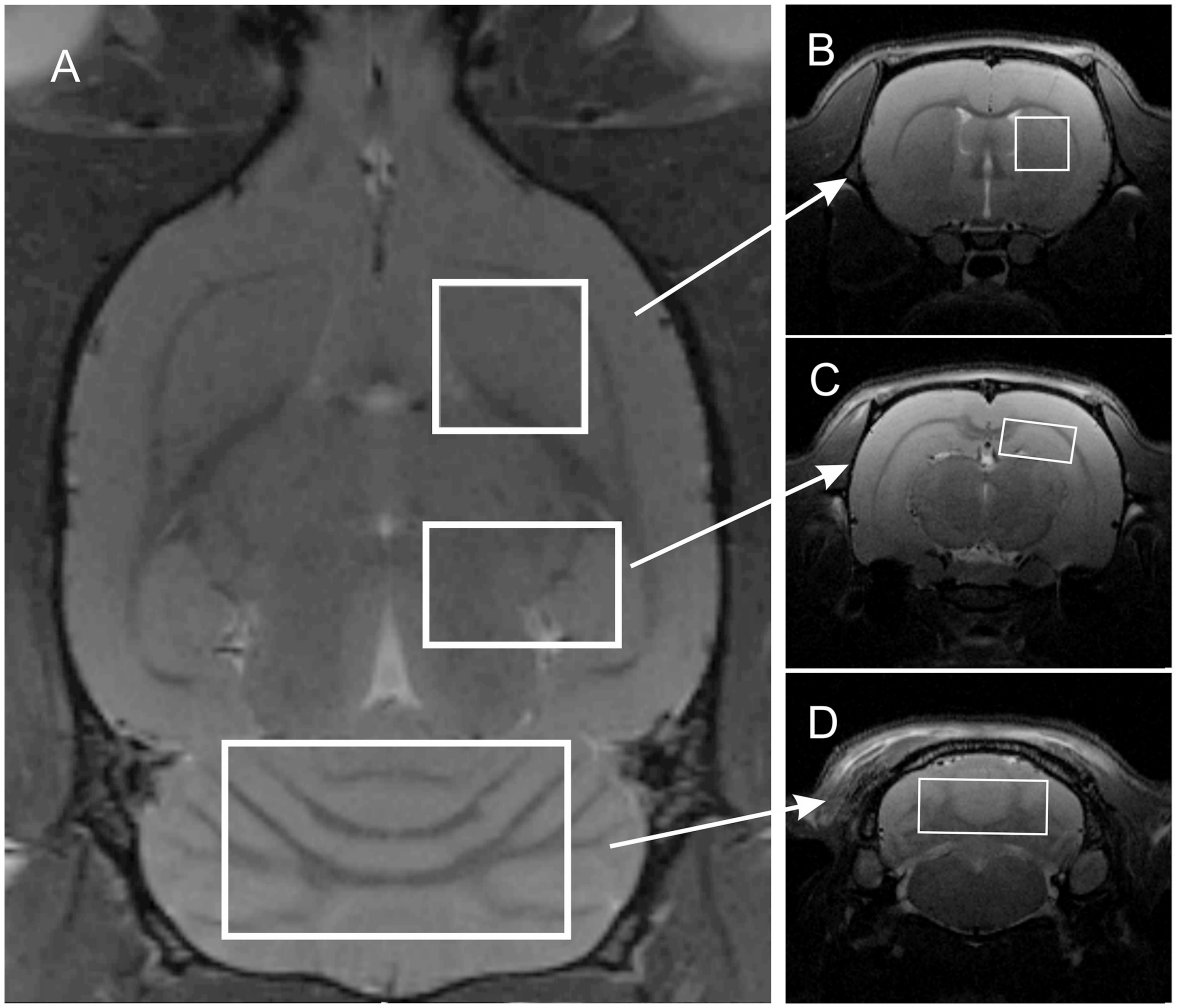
Localization of three volumes of interest on T2-weighted images: (A) axial slice; (B–D) coronal slices centered on the right striatum, right hippocampus, and cerebellum, respectively.
